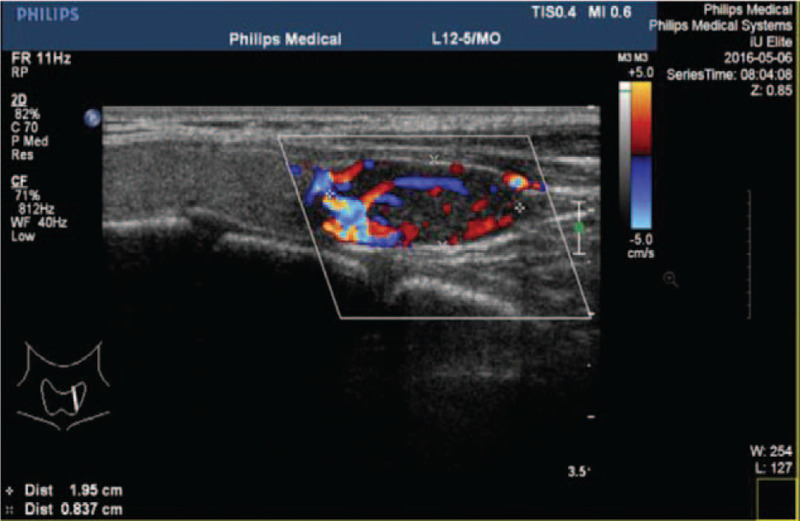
Ultrasound of the patient showed a 22.4 mm × 7.8 mm solid single nodule localized at the left lower lope of thyroid gland.
